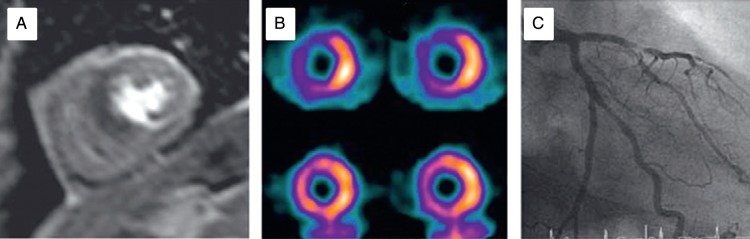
Imaging in chronic ischaemia: (*A*) cardiac magnetic resonance first pass perfusion demonstrating mid-ventricular anterior and septal abnormality. (*B*) Single photon emission computed tomography demonstrating the anterior and septal stress (top row) abnormality that resolves at rest. (*C*) Angiography in the same patient showing severe left anterior descending stenosis. Adapted from Greenwood *et al.* Cardiovascular magnetic resonance and single photon emission computed tomography for diagnosis of coronary heart disease: a prospective trial.^143^
